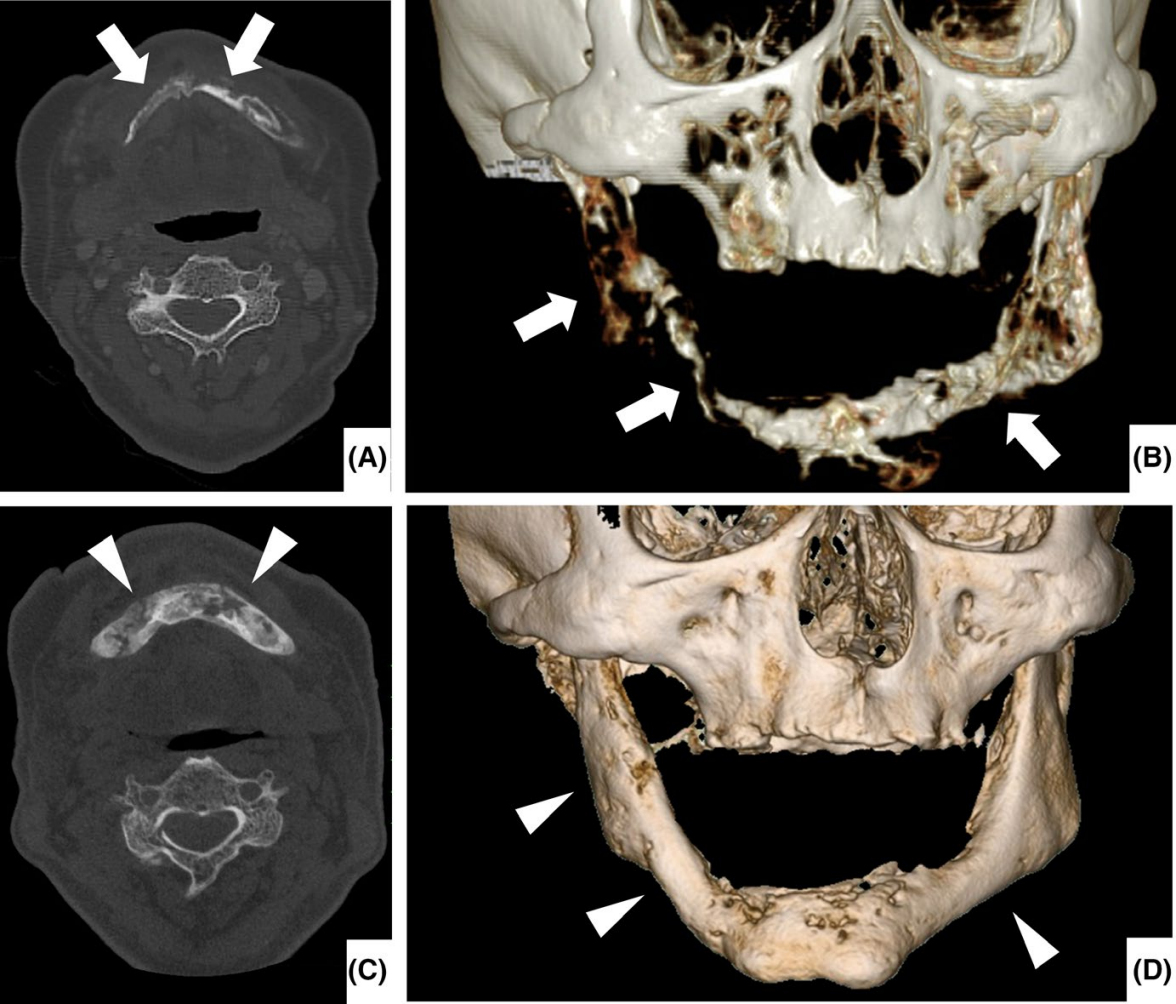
Facial computed tomography performed (A, B) on the first visit to our department and (C, D) 4 mo later. A, Cross‐sectional image shows sclerosing changes around marked osteolytic changes on both sides of the mandibular bones (arrows). B, Reconstructed image more clearly shows osteolytic changes on both sides of the mandibular bones (arrows). C, Cross‐sectional image shows marked improvement of sclerosing and osteolytic changes (arrowheads). D, Reconstructed image shows marked regeneration of the mandibular bones (arrowheads)
